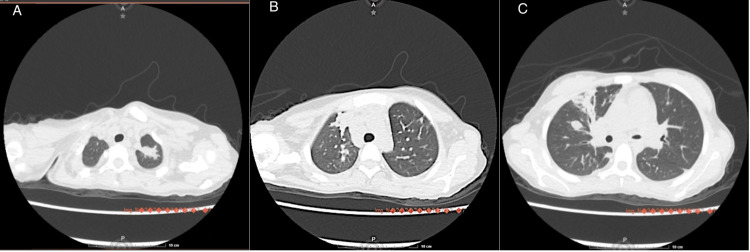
Computed tomography of the chest A) Axial slice at the level of the lung apices showing a parahilar lesion. B) Axial slice at the level of the middle lobe showing diffuse bilateral subsolid nodules. C) Consolidation of the posterior basal segment of the left lower lung lobe.
